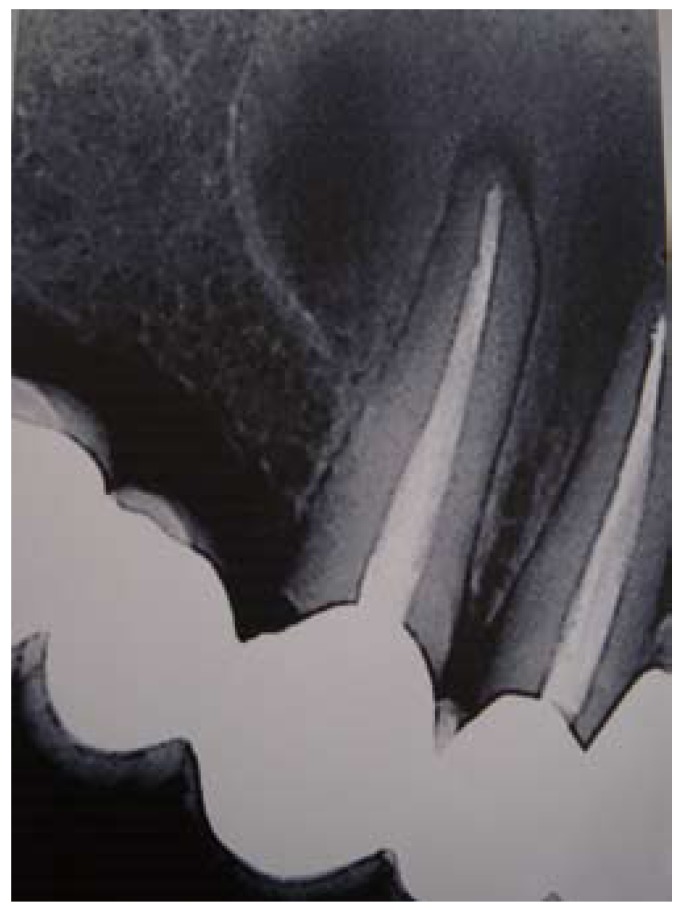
Periapical X-ray view. Radiotransparency in the anterior zone of the right upper maxilla, enveloping teeth #6, #7 and #8.
